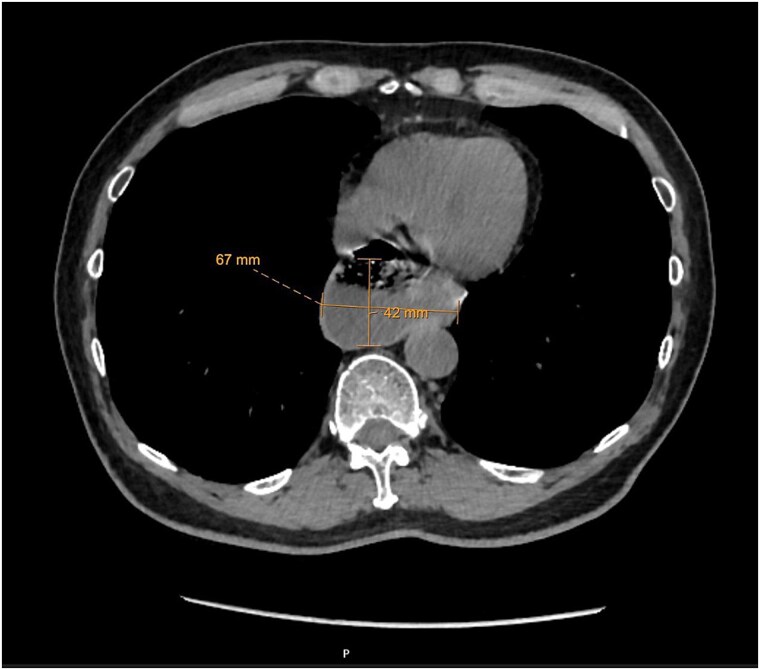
Axial CT demonstrating a 5 × 7 cm distal esophageal diverticulum with a 5-cm hiatal hernia.
